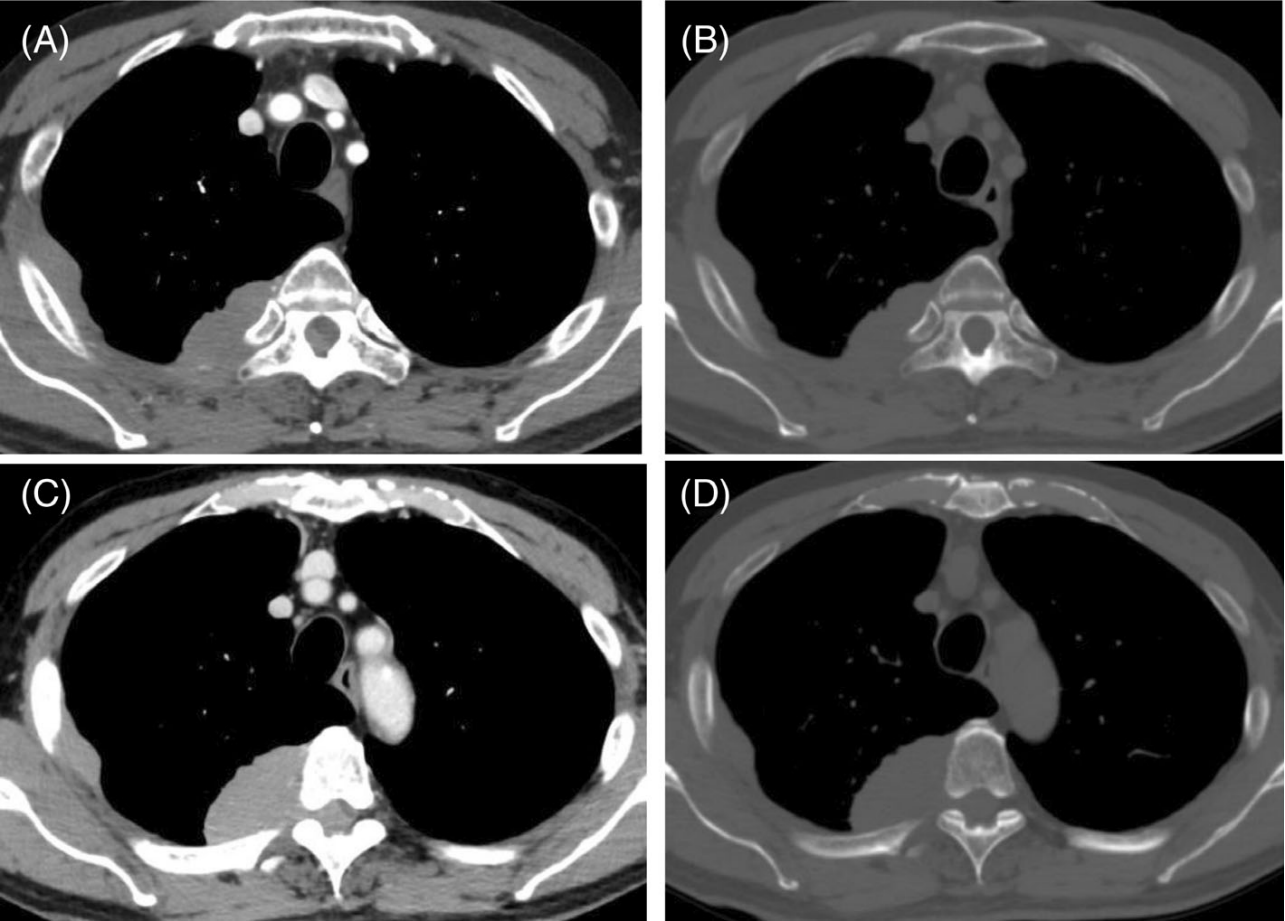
(A, B) A chest computed tomography (CT) scan showing a right chest wall mass spreading along the rib, without bone destruction, with nodular pleural thickening in the right lateral chest. The mass showing homogeneous soft tissue density, without calcification and low‐density area. (C, D) CT scan showing a mass extending from the thoracic cavity to the spinal canal at intervertebral foramen, called ‘dumbbell‐shaped lesion’.
